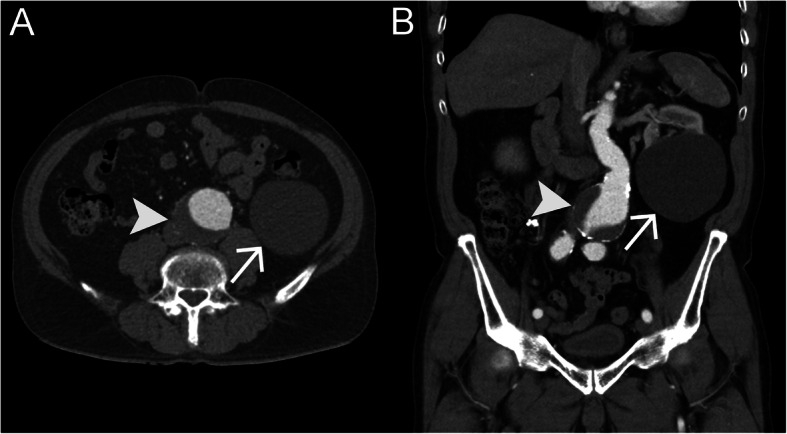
Simple renal cyst detected in a CT scan. Contrast-enhanced CT scan of the abdomen in axial (a) and coronal (b) plane, arterial phase, demonstrates an AAA (arrowhead) with mural thrombus and patent lumen and a large hypodense mass on the lower left renal pole, representing a simple renal cyst (arrow)
